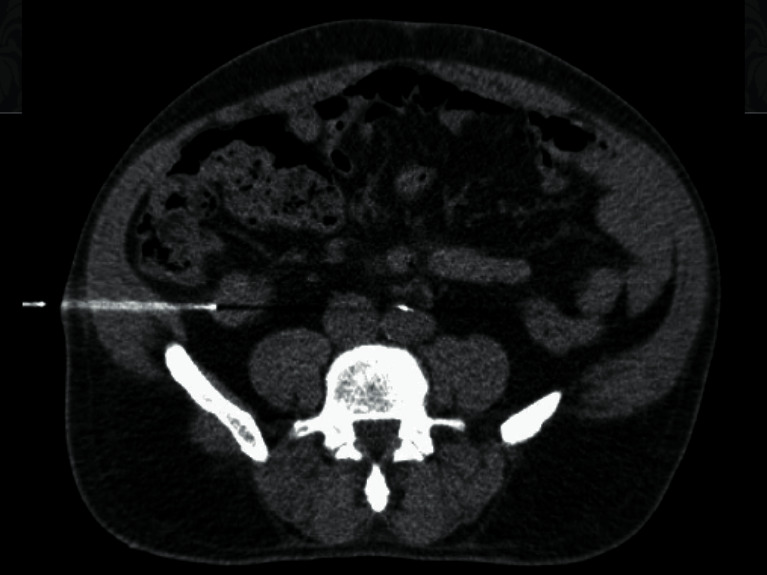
CT-guided core needle biopsy of the appendiceal mass.
